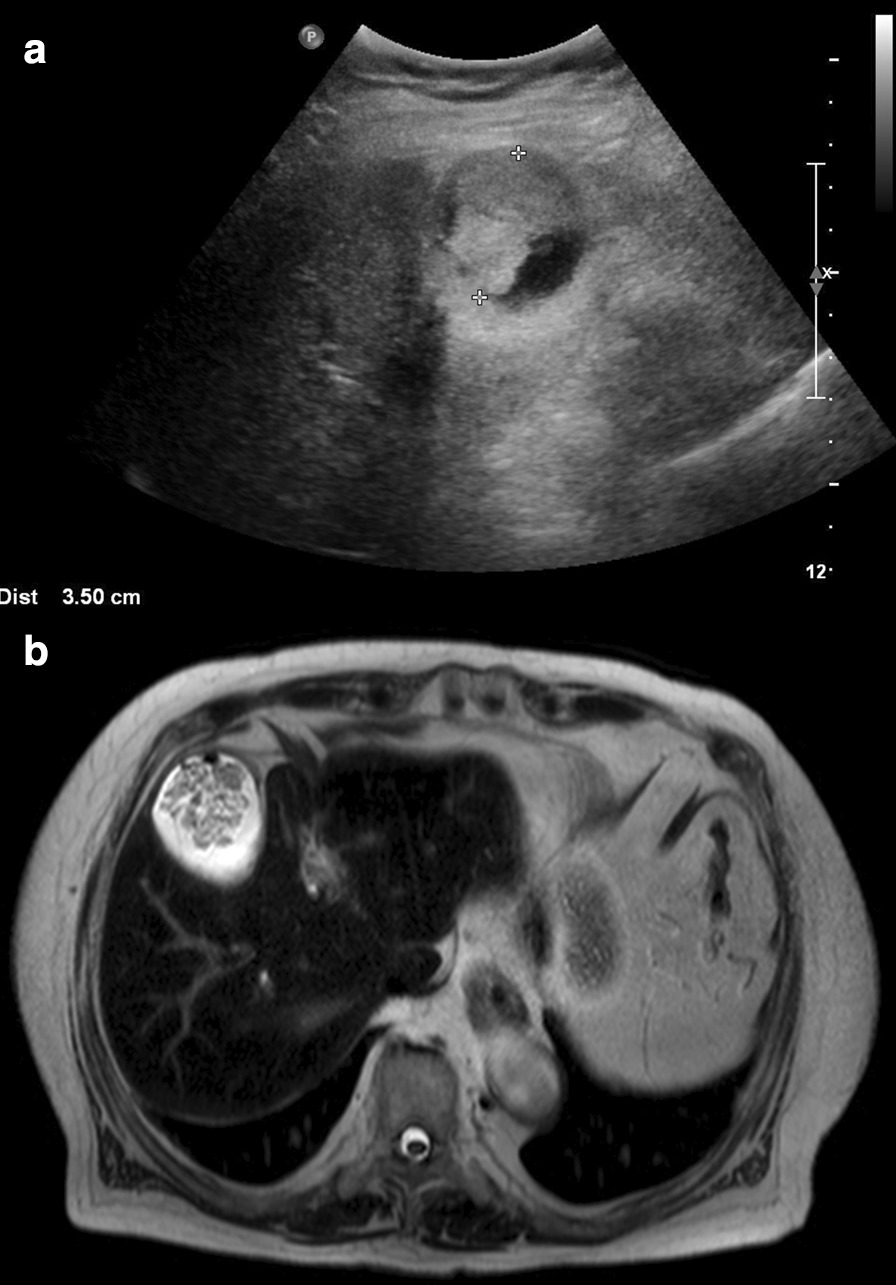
a Transabdominal sonography findings. The gallbladder is almost completely filled with a papillary mass. b Magnetic resonance imaging findings. T2-weighted magnetic resonance imaging shows a papillary mass within the gallbladder. There is no evidence of gallbladder wall or liver invasion
